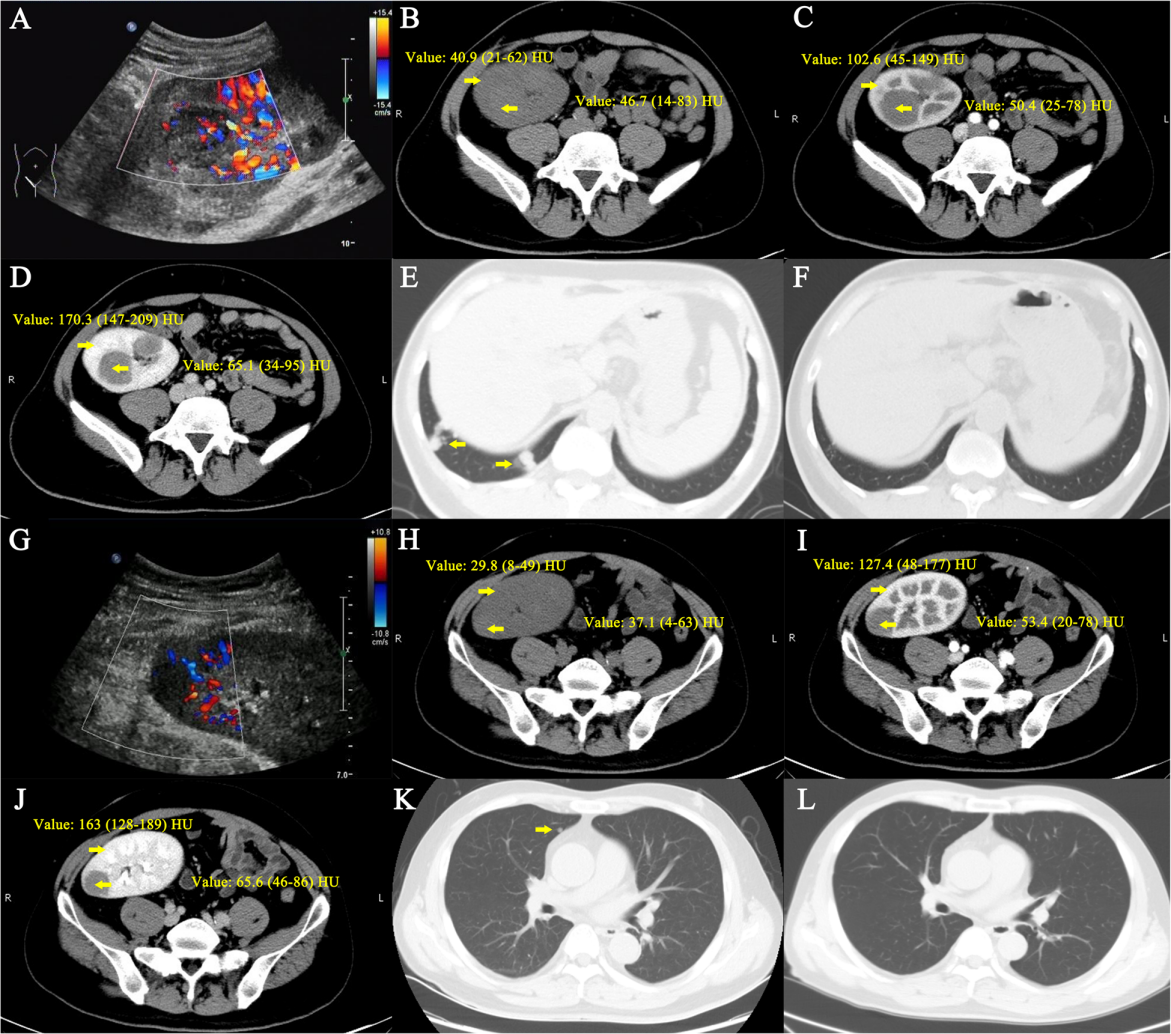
US and CT manifestations. Images A–F and G–L are from recipients 2 and 3, respectively. A and G: Color Doppler flow images revealed solid hypo-echo neoplasm with punctiform blood flow signals in the transplanted kidneys. B and H: Non-enhancing CT revealed slightly higher densities of neoplasms than allografts. C, D, I, J: Enhanced multiphase images revealed slow and relatively homogeneous enhancement inside the neoplasms, with lower densities than the allografts. E and K: CT revealed pulmonary metastases. F and L: CT revealed elimination of metastases after targeted therapy
